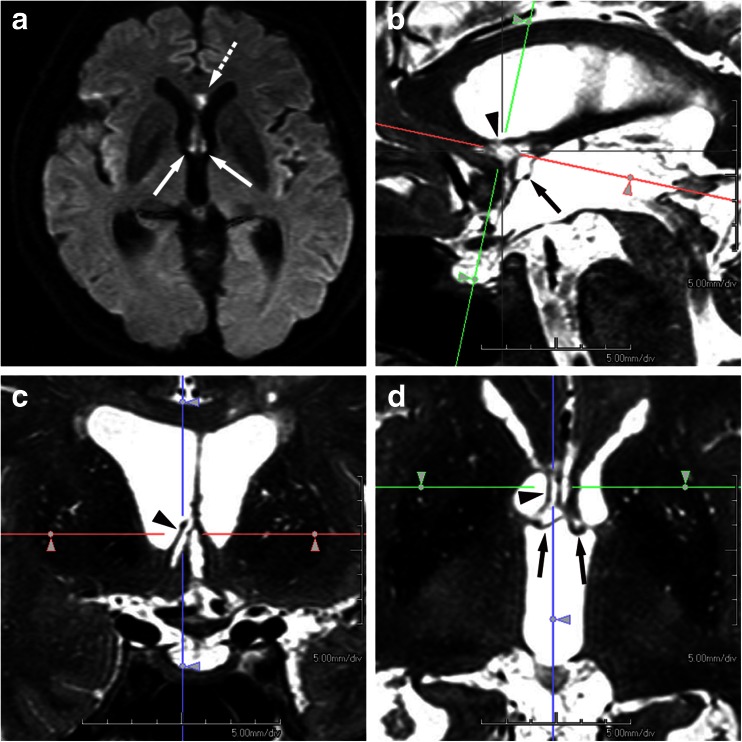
A 67-year-old man with a ruptured aneurysm of the ACoA was treated by transarterial coil embolization on the day of onset. Disorientation was suspected on the next day of embolization, but formal neuropsychological assessment was hardly valid due to confusional state in the acute phase. Diffusion-weighted image performed on the 4th day of embolization (a) demonstrates acute infarction in the column of fornix, bilaterally (*arrows*) and in the rostrum of the corpus callosum (*dashed arrow*). Two months later, formal neuropsychological tests revealed amnesia, confabulation, and disorientation. Paramedian sagittal (b), coronal (c), and axial (d) MPR images were generated from 3D data of T2-weighted volumetric isotropic turbo spin-echo acquisition (VISTA). The coronal image (c) corresponds to the *green line* in the paramedian sagittal (b), and the axial image (d) corresponds to the *red line* in the paramedian sagittal (b), so that both images pass through the superior part of the paraterminal gyrus in the right (*arrowheads* in b–d). Infarcted foci involve not only the pars libera of the column of the fornix bilaterally (*arrows* in d) and the rostrum of the corpus callosum (not shown) but also the paraterminal gyrus in the right and the anterior commissure (*arrow* in b). Thus, it may well be said that the involvement of the paraterminal gyrus and the anterior commissure found on 3D images in the chronic phase had not been visualized on the initial 2D diffusion-weighted images in the acute phase
